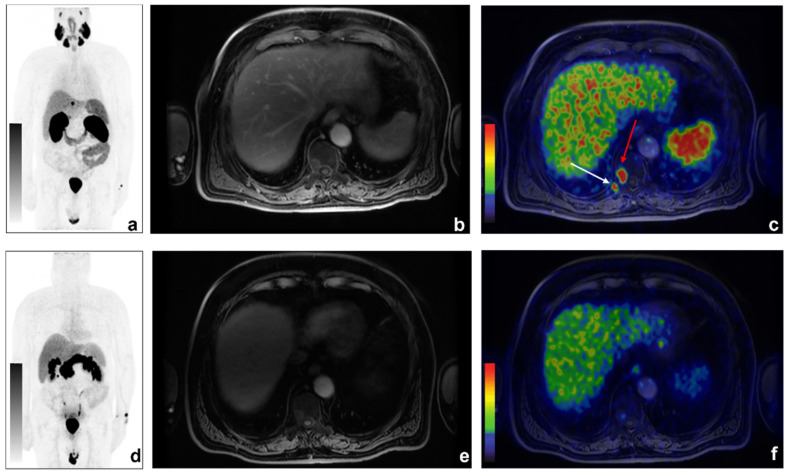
An example of discordant findings between ^68^Ga-PSMA and ^68^Ga-DOTA-RM2 PET/MRI in bone metastases detection. A 70-year-old patient previously treated with radical prostatectomy for PCa (Gleason 5 + 4; pT3aN0) experienced biochemical recurrence of PCa (PSA = 2.16 ng/mL). ^68^Ga-PSMA PET/MRI showed radiotracer uptake in correspondence of right D10 hemisome ((a): ^68^Ga-PSMA MIP; (b): axial LAVA-FLEX MRI; (c): axial ^68^Ga-PSMA PET/MRI, red arrow) and the right posterior tract of the X rib (c): white arrow). Non-significant uptake was detected on ^68^Ga-DOTA-RM2 PET/MR images (d): ^68^Ga-DOTA-RM2 MIP; (e): axial LAVA-FLEX MRI; (f): ^68^Ga-DOTA-RM2 PET/MRI).
